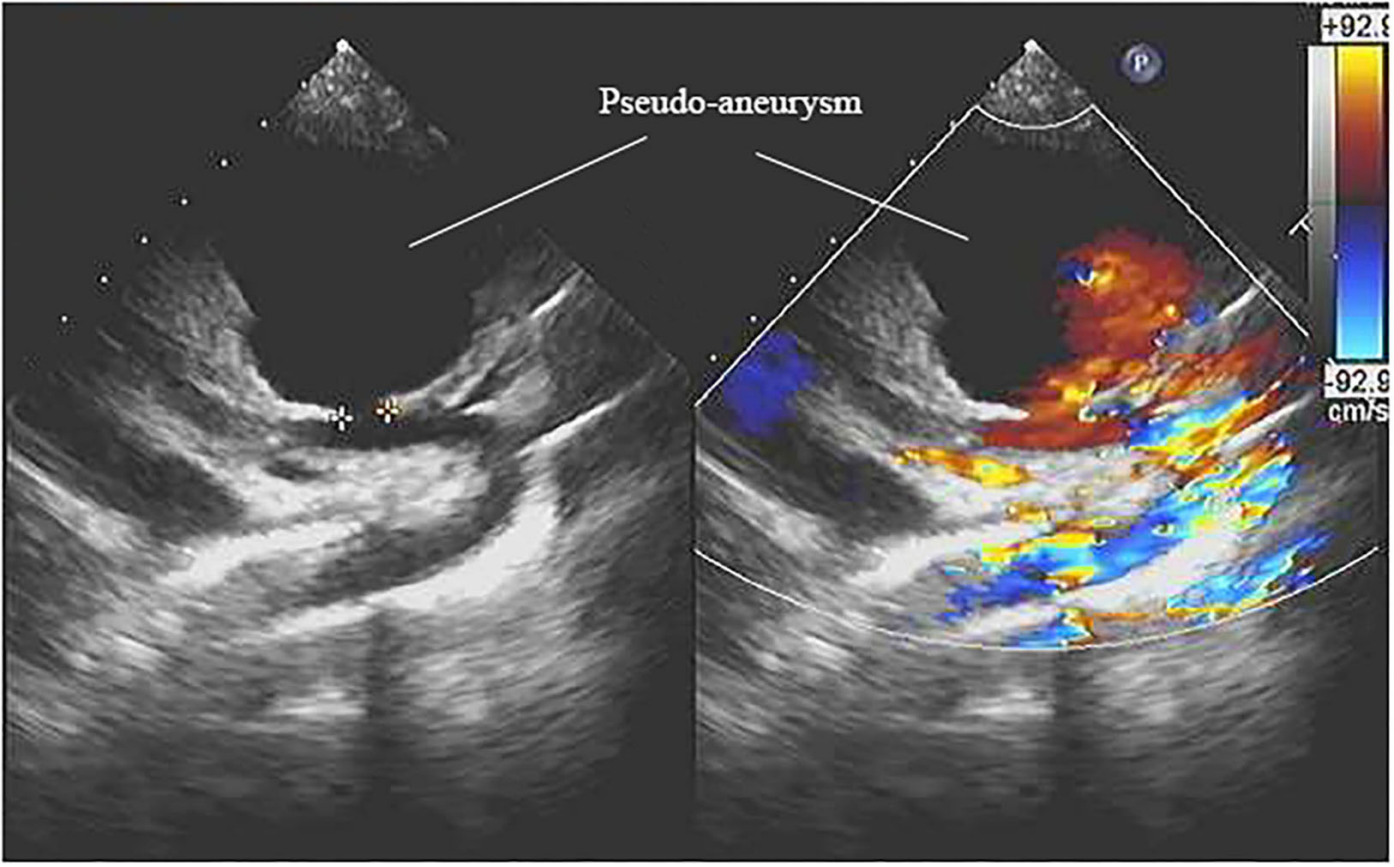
An echocardiography view represents the aortic pseudo-aneurysm.
